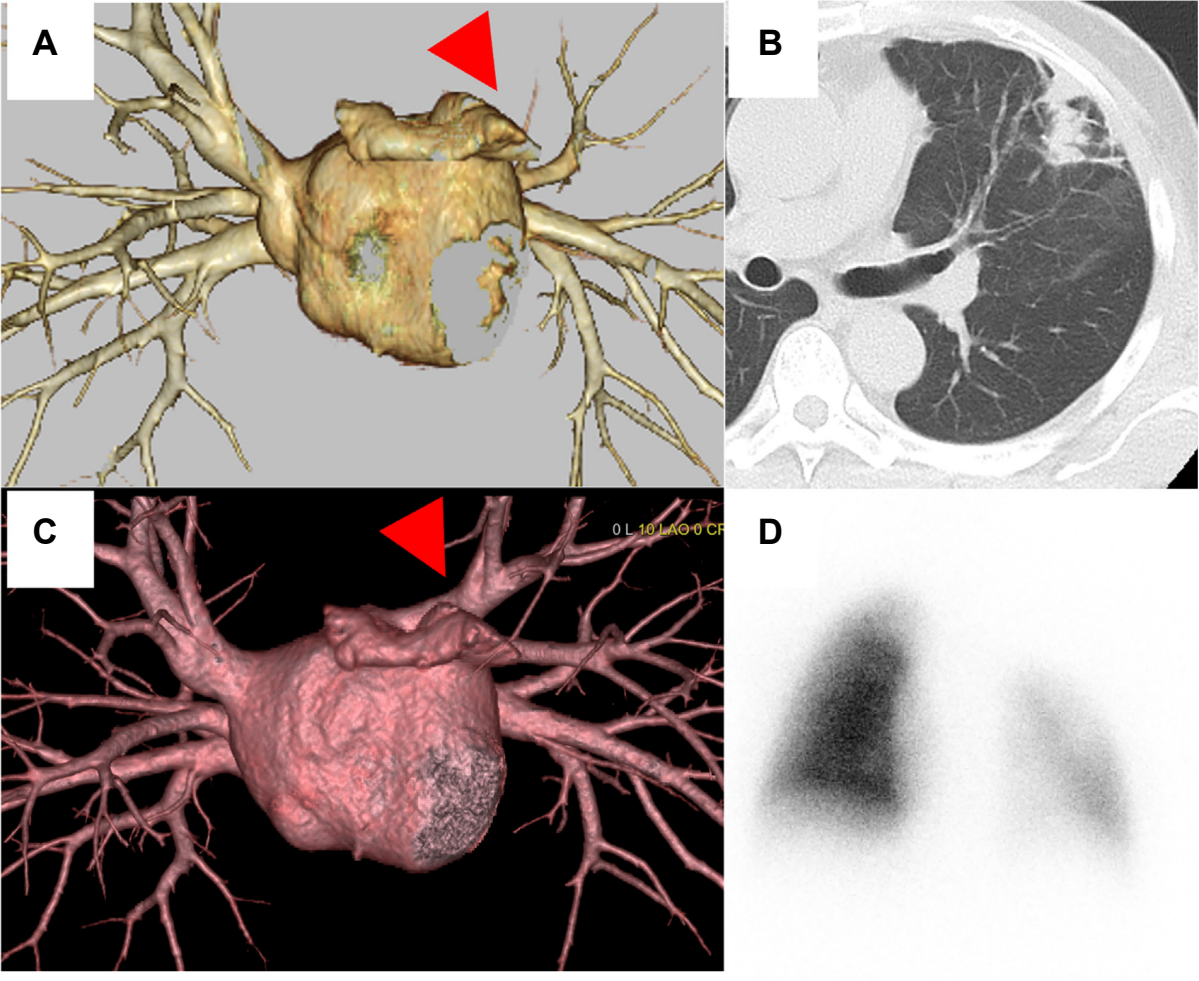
Preoperative image findings. Three-dimensional CT scans (A) before and (B) after catheter ablation. A shadow of the left upper pulmonary vein (red arrowheads) was absent. (C) Computed tomography findings in the lung revealed the consolidation with ground glass shadow in the left upper lung. (D) Pulmonary perfusion scintigraphy showed the reduced blood flow in the left upper lung.
